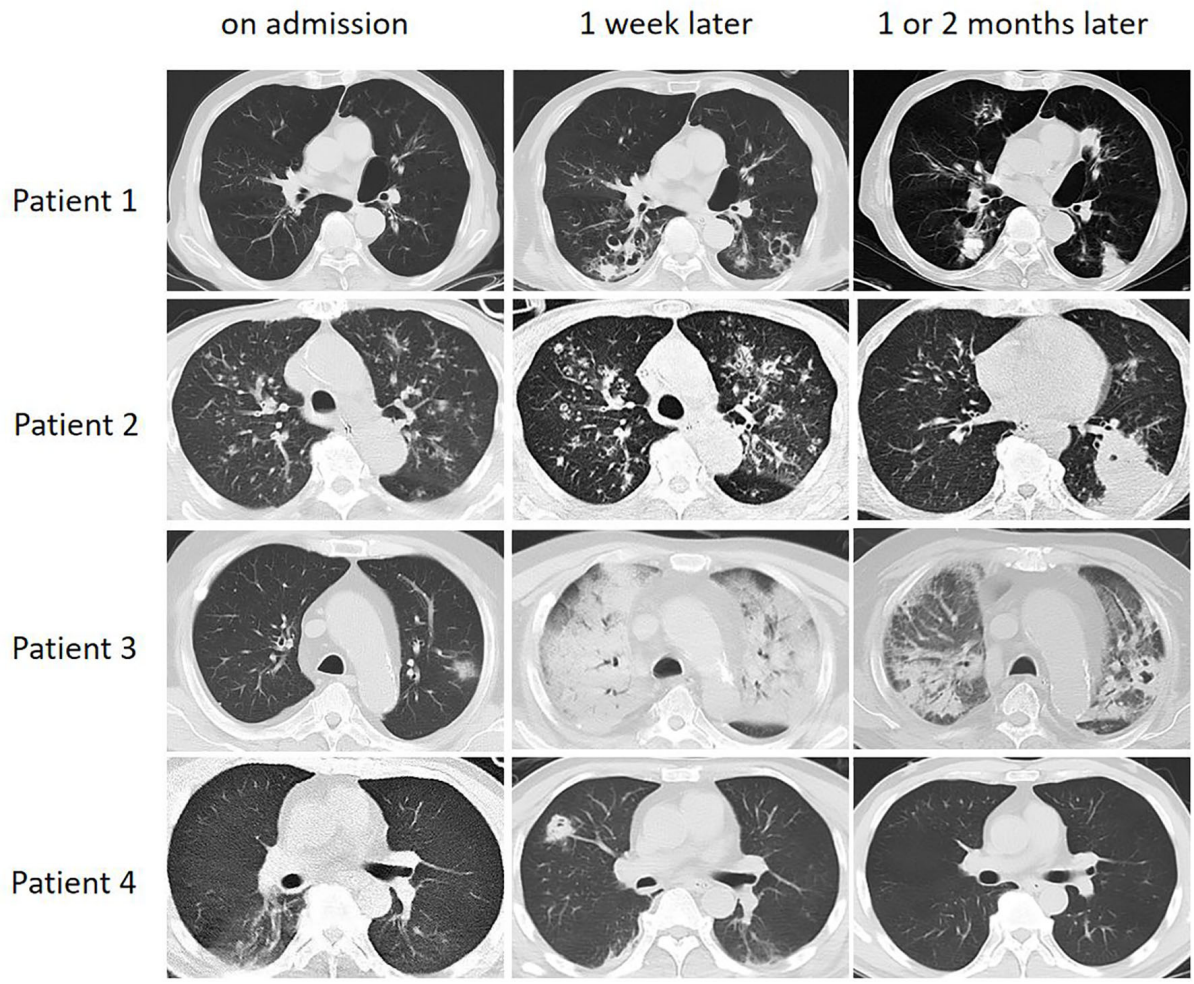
Serial CT imaging of four representative non-neutropenic IPA cases. • Patient 1: A 62-year-old male with COPD. The initial CT showed bronchial wall thickening, followed 1 month later by the development of multiple thin-walled cavities and wedge-shaped consolidation. • Patient 2: A 70-year-old male with COPD and influenza infection. The initial CT revealed a tree-in-bud pattern. One month later, a new consolidation with a small cavity appeared in the dorsal side of the left lung lobe. • Patient 3: A 60-year-old male with diabetes and severe viral pneumonia. CT at 1-week post-admission demonstrated diffuse bilateral consolidation, which subsequently evolved into multiple thick-walled cavities. • Patient 4: A 53-year-old male with diabetes as the sole risk factor. CT performed 1 week after admission showed isolated nodules, which had resolved completely on follow-up imaging 2 months later.
